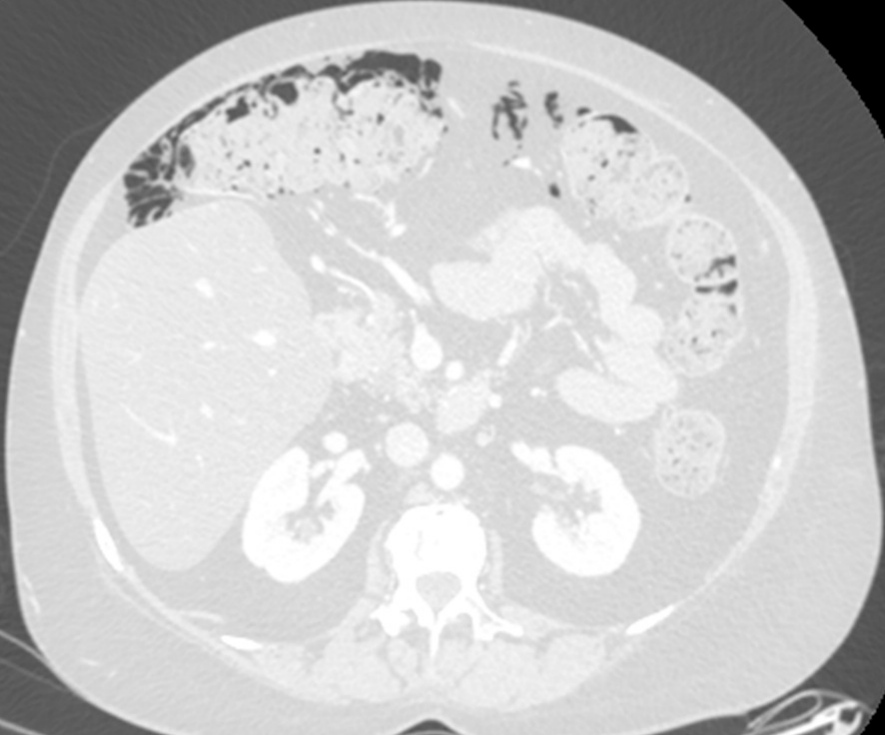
Case 2. A 52-year-old female with metastatic (lymph nodes, bone and brain) breast cancer, receiving chemotherapy and corticosteroids, submitted to body CT scan to rule out abdominal injury; axial CT image (detail of transverse colon), lung window. The image shows a moderate amount of intramural gas involving the caecum, ascending and transverse colon, with an extensive amount of gas in the mesenteric and pericolic fat; haustra are regular.
